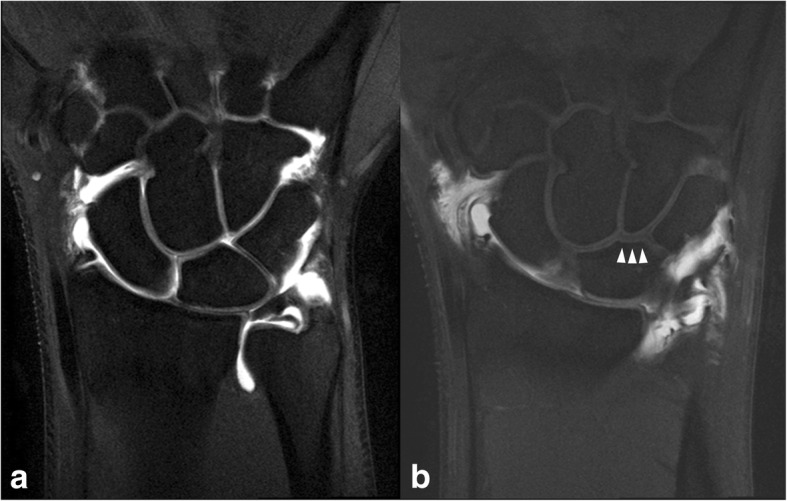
Examples of the detection of a medial hamate facet of the lunate on magnetic resonance arthrography. Coronal fat-saturated, turbo spin-echo, T1-weighted arthrographic images of (a) Type I lunate and (b) Type II lunate with a medial hamate facet (arrowhead)
